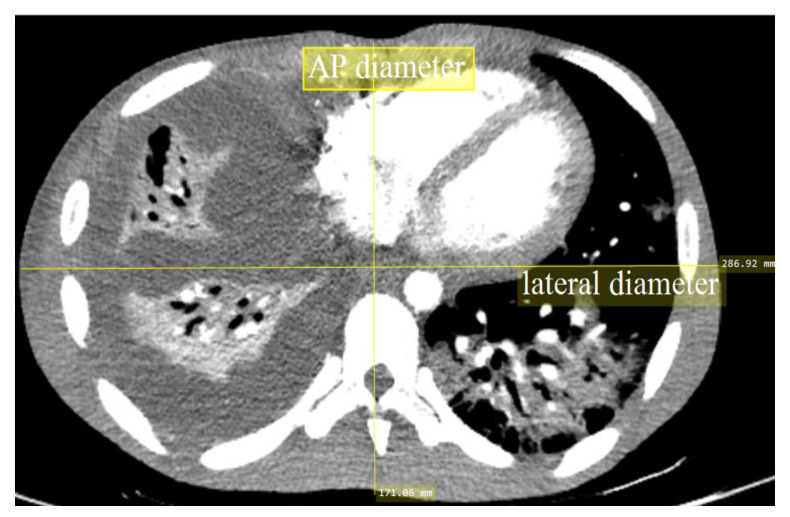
A patient’s anteroposterior (AP) measurement at the mid-slice of the 3D computed tomography (CT) images.
